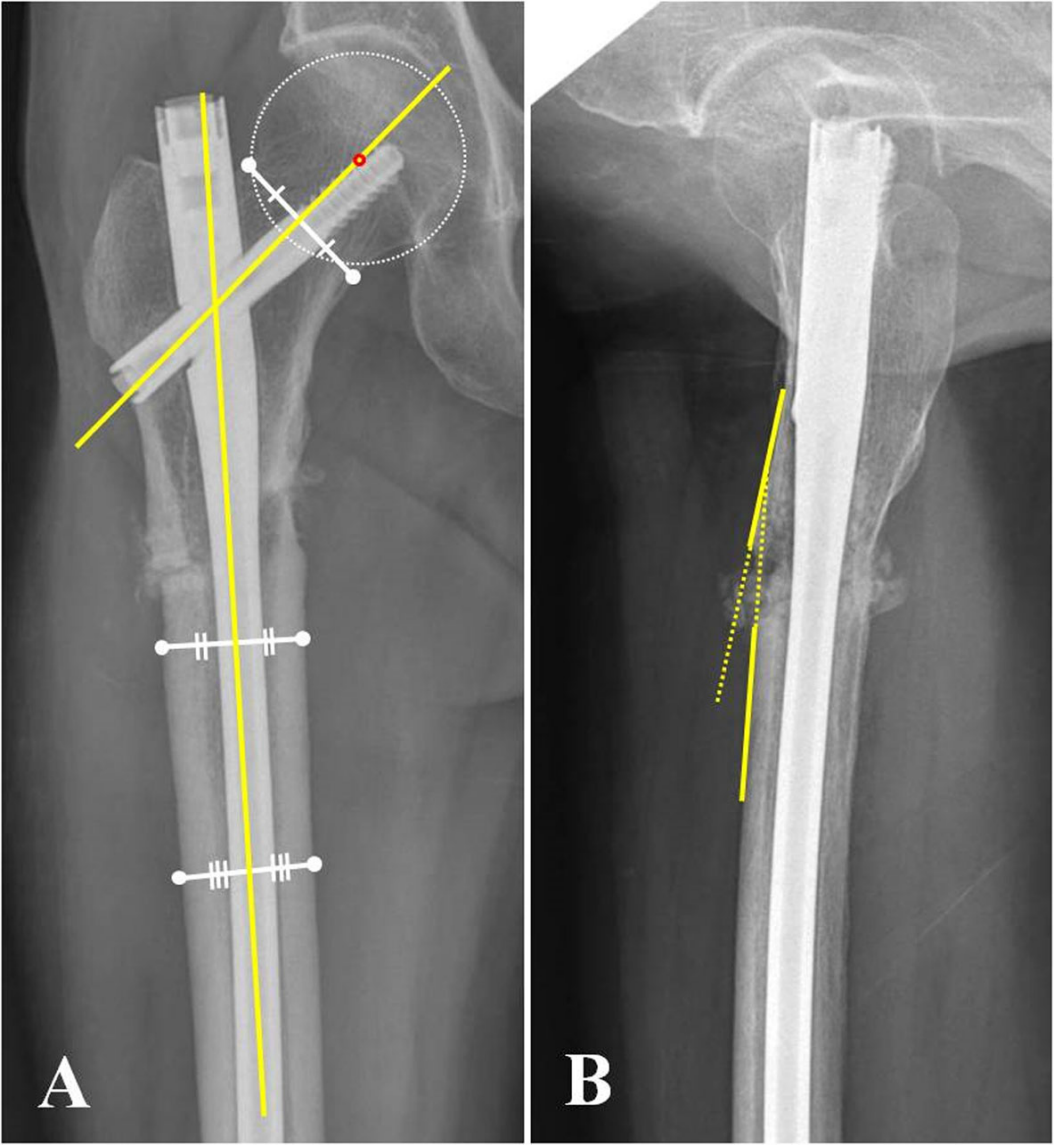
Radiographic measurements of the neck-shaft angle (a) at the anteroposterior radiograph and sagittal anterior angulation (b) at the lateral radiograph
